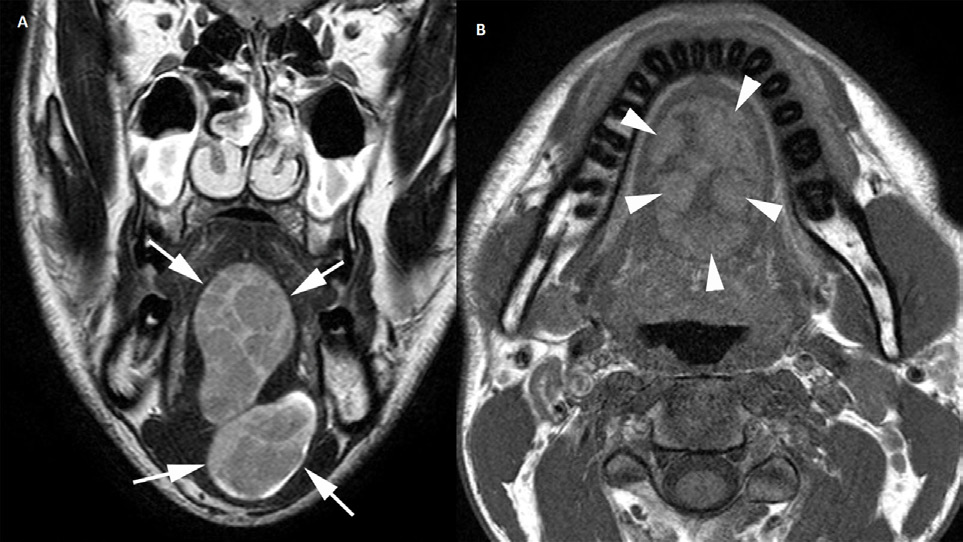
Coronal T2-weighted image (A) depicts well-demarcated, bilobular heterogeneous mass lesion (arrows) in the midline of the tongue, extending in the floor of the mouth. The plain T1-weighted image (B) shows spontaneous hyperintense areas (arrowheads) within the lesion, indicating the presence of fat.
